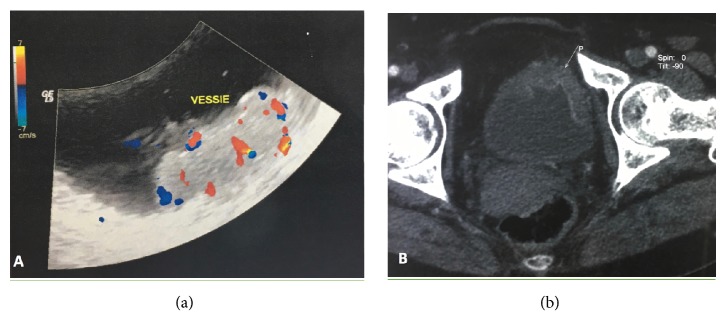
Preoperative imaging of the bladder lesion. (a) Ultrasonography showing vascularized parietal lesion. (b) Scanographic aspect of the bladder tumor.
